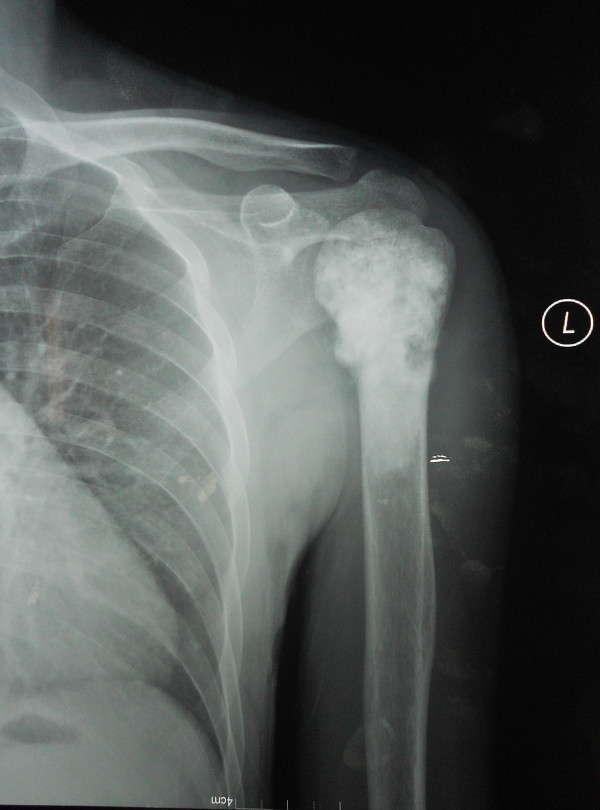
Preoperative X-ray (AP view) of a 39-year male patient with left humeral osteosarcoma.
